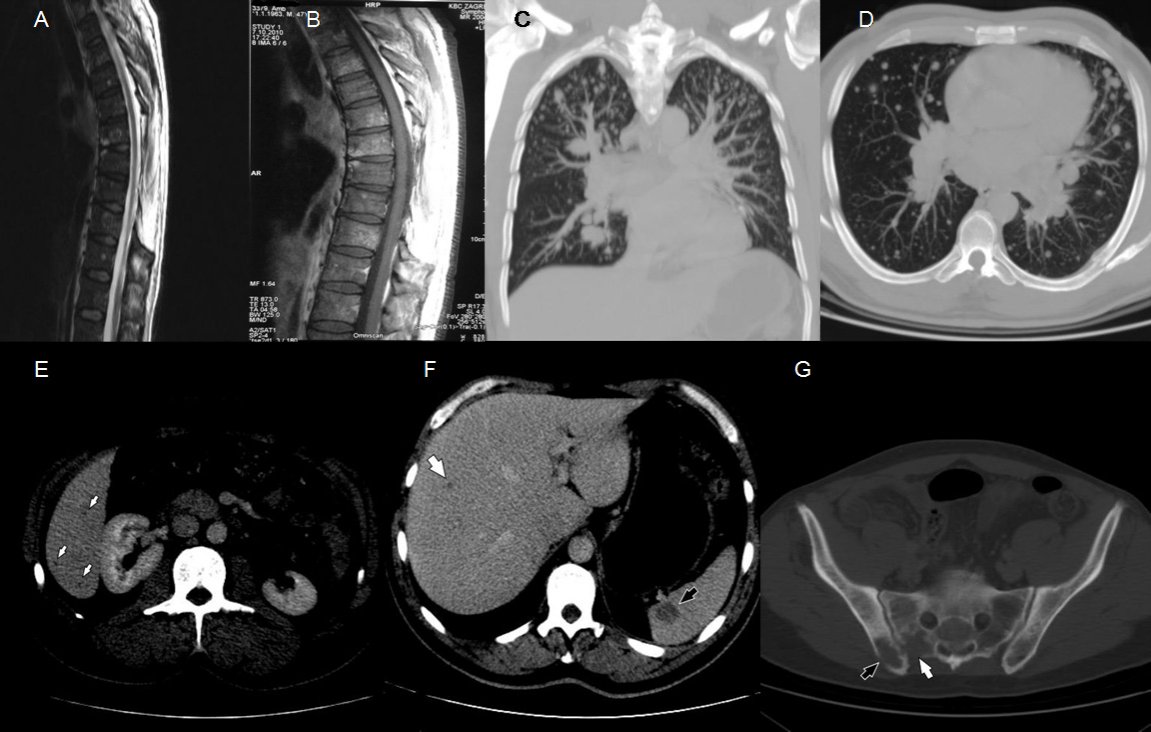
Radiological evaluation. Magnetic resonance sagittal T2 weighted image (image A) and postcontrast sagittal T1 image (image B) of the thoracic spine shows millimetric hyperintense metastases with no residual-recurrent tumor. Multislice computed tomography of the chest and abdomen demonstrated osteolytic (image G) and parenchymal metastases (arrows) in lungs (images C and D), liver and spleen (images E and F).
